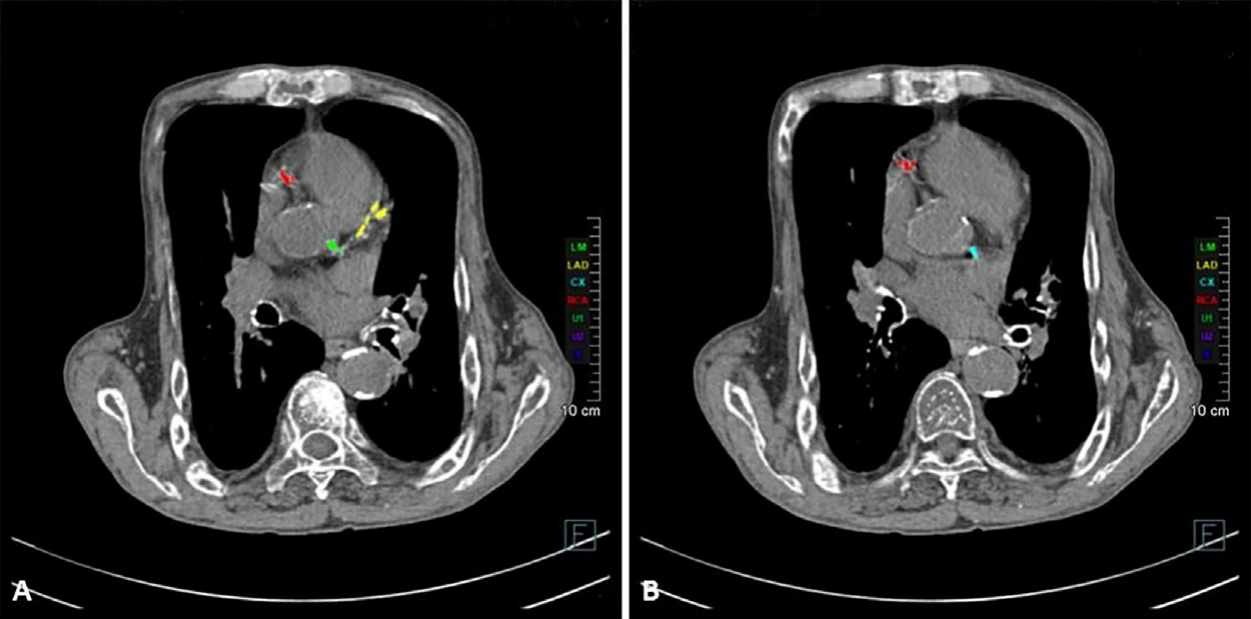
Assessment of coronary calcium score by cardiac CT. The cardiac CT revealed thoracic deformity, vascular and bronchial calcification. (A) Green: left main artery (LMA). Red: the right main artery (RCA). Yellow: left anterior descending artery (LAD). (B) Blue: left circumflex artery (CX).
